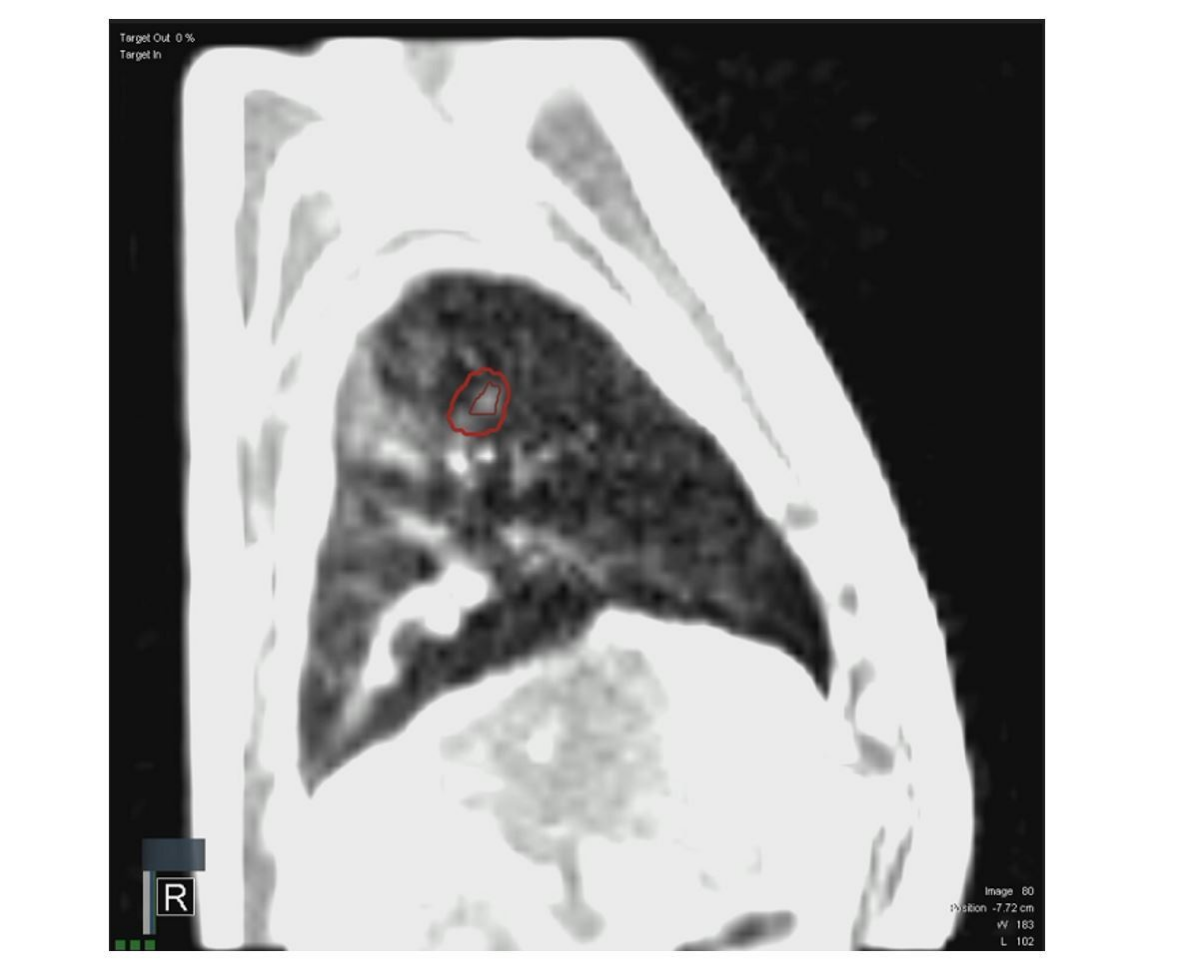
Real-time tracking of 0.89 cm3 gross target volume with 5.0 mm boundary was feasible on clinical magnetic resonance-guided radiotherapy system, minimizing potential geometric miss observed from auto-match of cone-beam computed tomography-based registration for patient. ​​​​​​​
